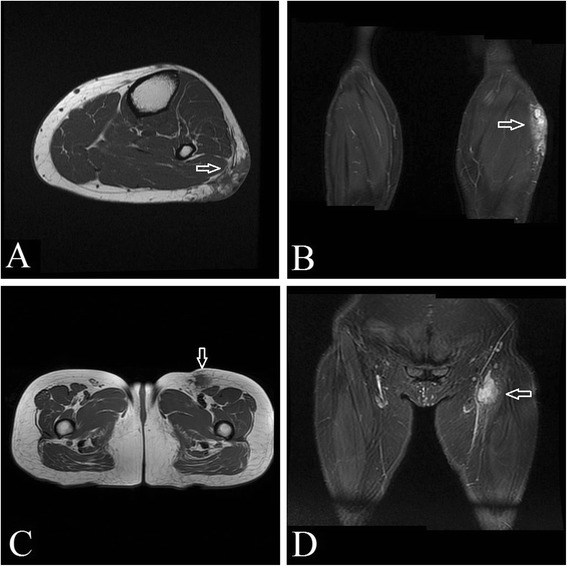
The imaging (MRI) of the tumor. MRI shows multiple subcutaneous nodules at the left calf below the knee integrating to a mass about 6 cm × 5 cm. The biggest one was about 1.4 cm × 0.9 cm. (a, T1-weighted image shows low signal intensity, ; b, T2-weighted image (STIR) shows high signal intensity, ). MRI also detected a subcutaneous mass about 3.2 cm × 3.4 cm at the left thigh near the inguinal area (c, T1-weighted image shows low signal intensity, ; d, T2-weighted image (STIR) shows high signal intensity, )
